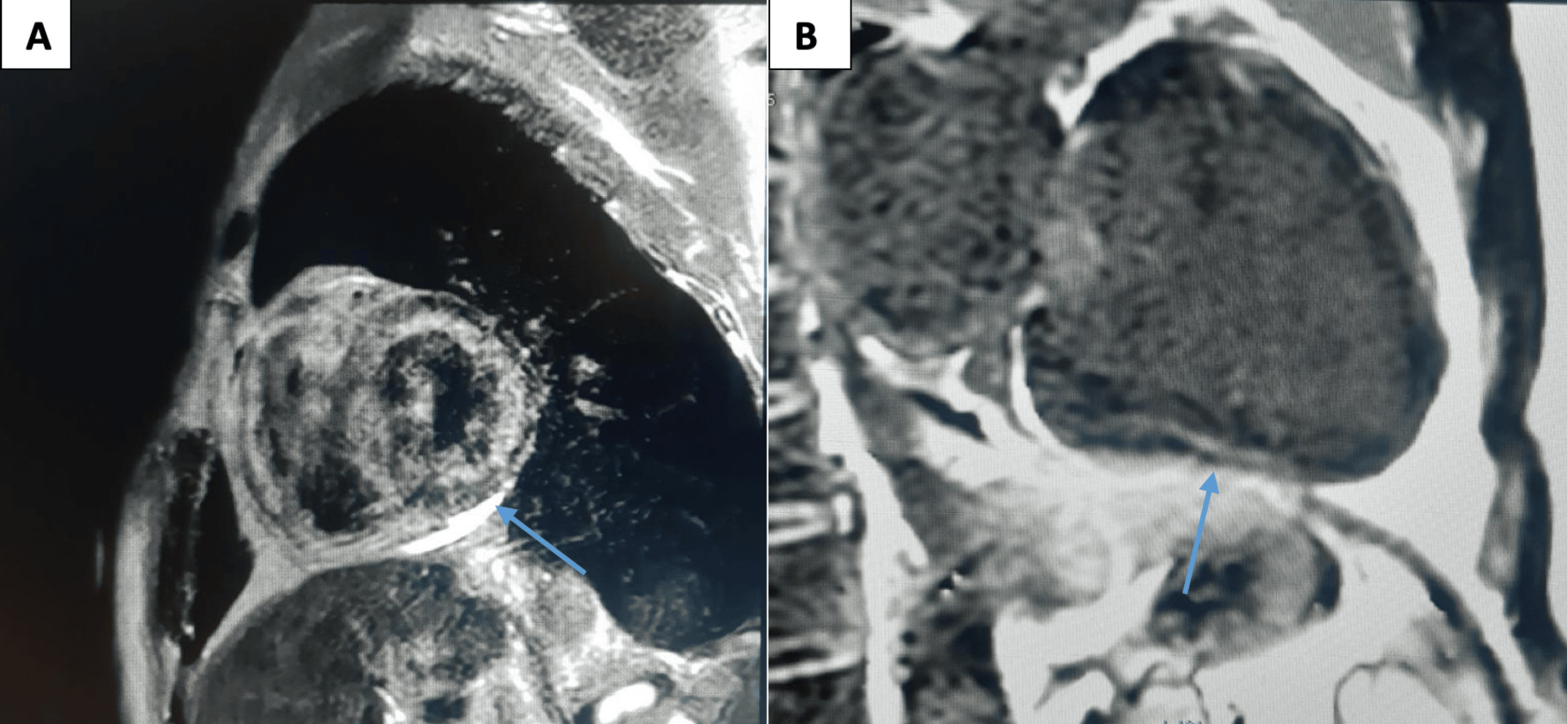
Cardiovascular MRI of the patient showing signs of myocarditis. (A) Short-axis T2-short-tau inversion recovery image showing high signal intensity in the lateral wall (blue arrow), consistent with myocardial edema. (B) Phase-sensitive inversion recovery sequence in long-axis view highlighting subepicardial late gadolinium enhancement in the inferolateral wall (blue arrow), consistent with non-ischemic myocardial injury.
